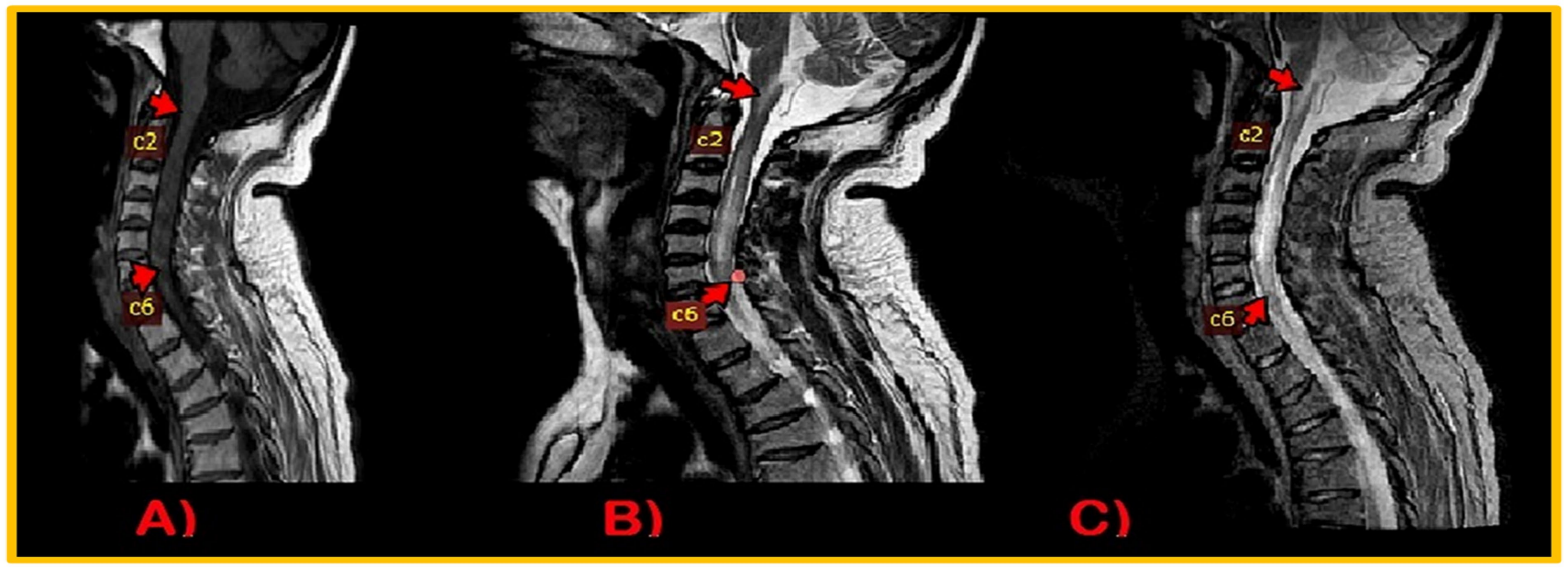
MRI Cervical Spinal cord: intramedullary altered intensity signals extending from craniocervical junction to C6 vertebral. T1WI, isointense (A); T2WI/STIR, heterogenous hyperintense (B,C).
